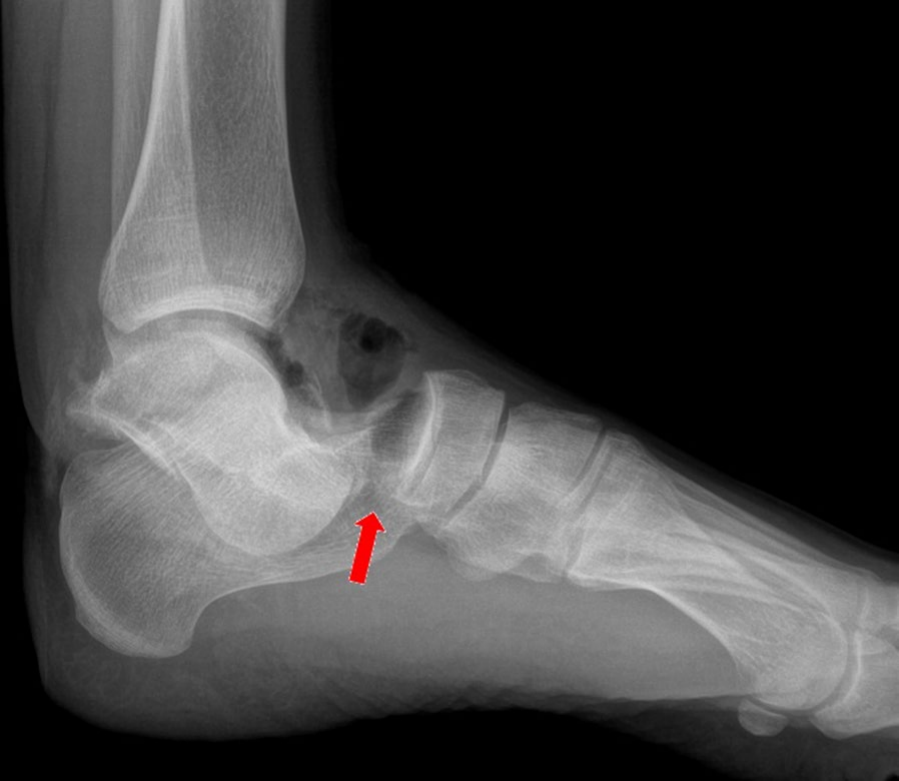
Latero-lateral X-ray showing a lateral subtalar dislocation. The red arrow shows inclusion of the posterior tibial tendon between the head of the talus and the navicular bone
